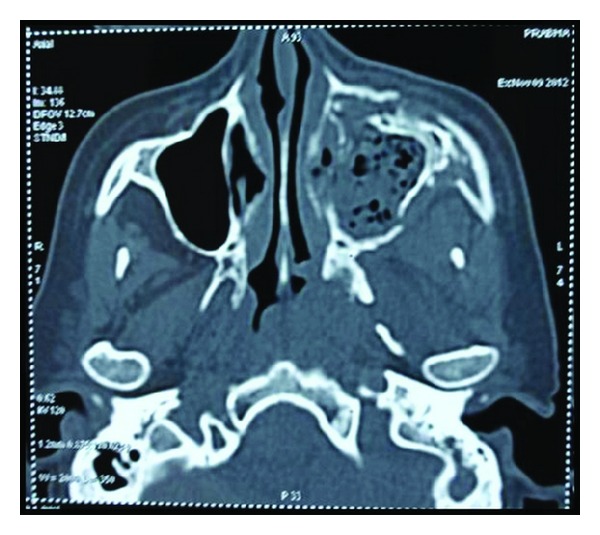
Axial view of CT showing destruction of posterior, medial, and anterior walls of left maxillary sinus.
